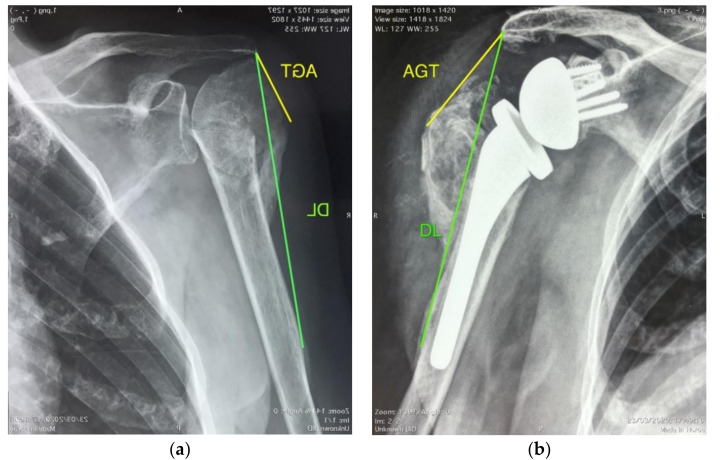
Radiographic acromion to greater tuberosity distance (AGT) and deltoid length (DL); (a) preoperative measurement; (b) postoperative measurement.
